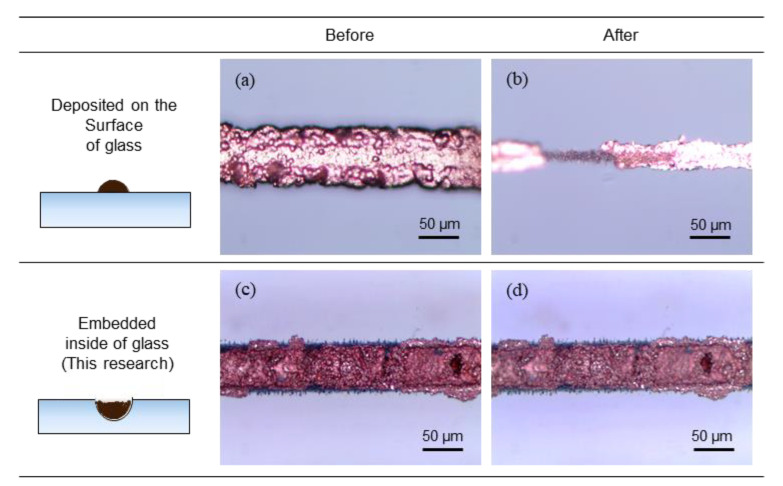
Durability test for deposited copper on the surface of the glass (a) before and (b) after the ultrasonic vibration test and inside channels; (c) before; and (d) after the ultrasonic vibration test.
